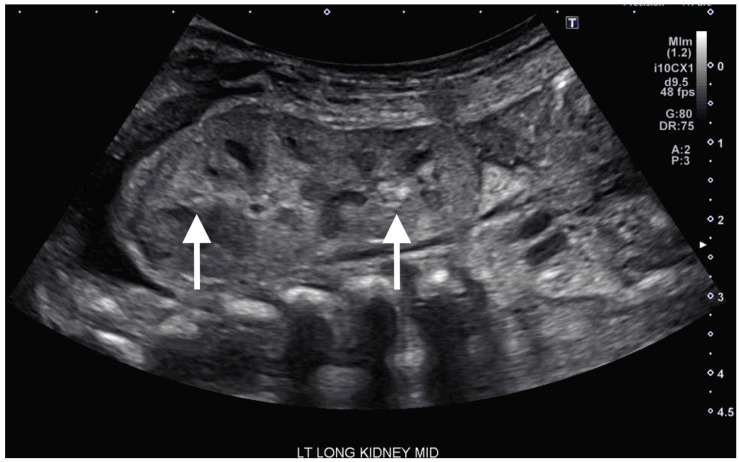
Ultrasound of left kidney on day of life 5 showing increased focal echogenicity of the upper and lower poles (arrows).
